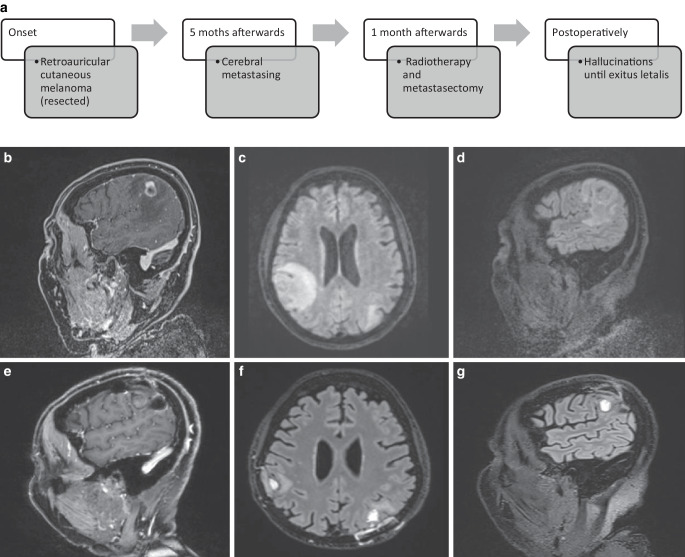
a Timeline case 2. b, c, d MRI scans at time of diagnosis. b The sagittal T1-weighted image shows an isointense lesion with a ring-like enhancement and perifocal edema. The fluid-attenuated inversion recovery (FLAIR)-weighted image in the axial plane (c) and sagittal plane (b) depicts a heterogeneously hyperintense space-occupying lesion in the right parietal lobe, surrounded by marked perifocal edema, and also a heterogeneously hyperintense lesion with minor perifocal edema in the inferior parietal gyrus on the left (c). There was no midline shift. e, f, g MRI scans 2 months after definitive radiosurgery of the right frontal and left parietal metastases and preoperative radiosurgery of the right parietal brain metastasis followed by osteoplastic craniotomy and resection, and 1 month after salvage left parietal metastasectomy. The sagittal T1-weighted contrast-enhanced (e) and T2-weighted fluid-attenuated inversion recovery (FLAIR) sequences axial (f) and sagittal images (g) show postoperative cavities with regression of the edema
